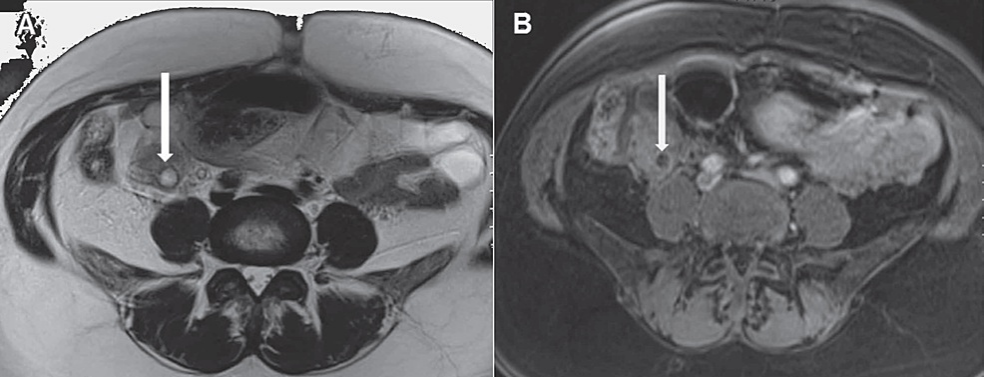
T1 and T2 weighted images (axial) (A) Axial T2-weighted image shows a rounded hyperintense structure (arrow) in the center of an ill-defined mass located at the ventral aspect of the right psoas muscle corresponds to thrombus formation within the right ovarian vein (B) Axial post-contrast T1 weighted image shows the lack of enhancement in the right ovarian vein (white arrow) due to thrombosis. From reference [13], with permission.
